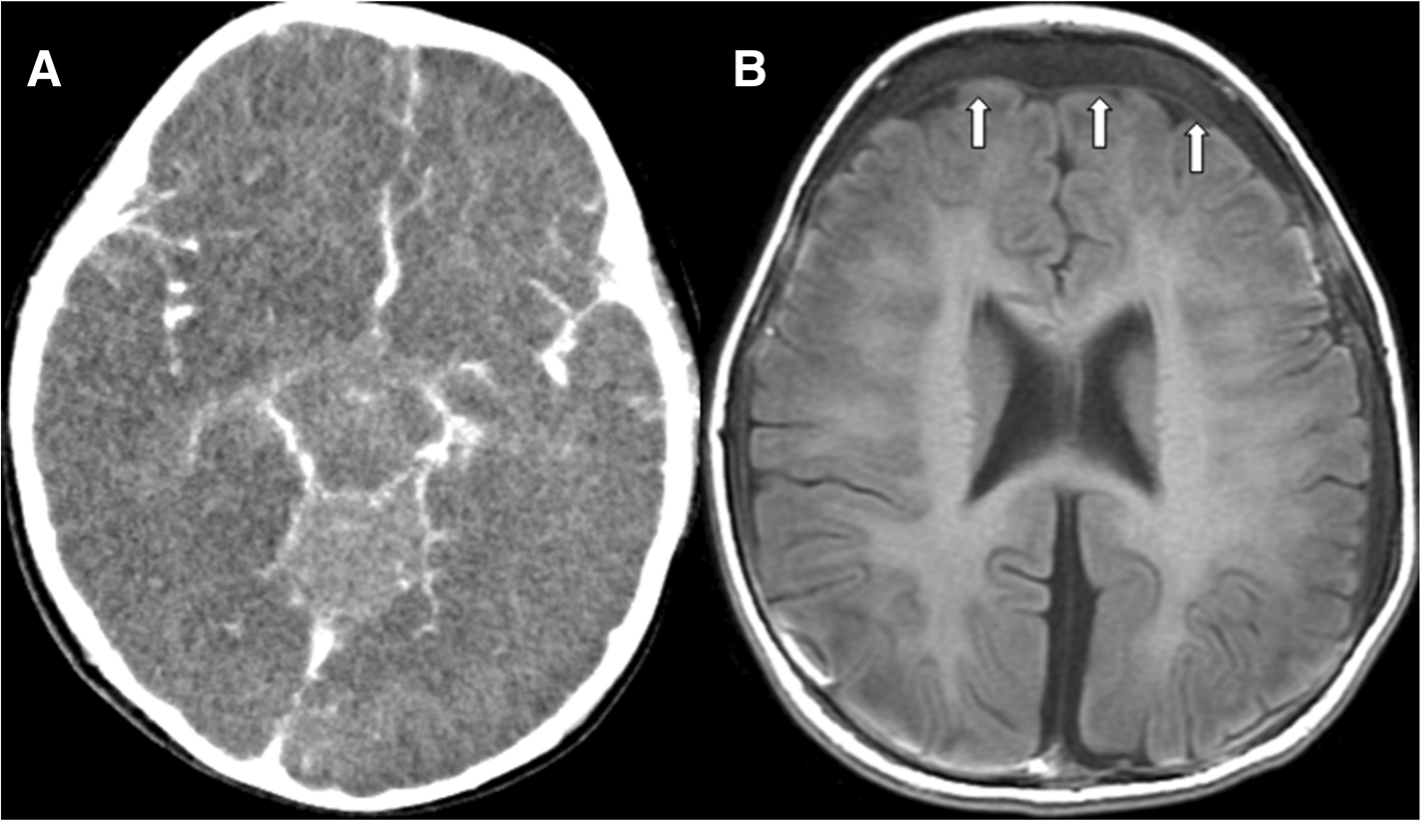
a. Brain CT at admission showed hypoxic-ischemic encephalopathy with severe brain edema; (b). Brain MRI (T1 flair) at day 14 showed subdural hemorrhage of different stages (white arrows) over bilateral fronto-parietal convexities
